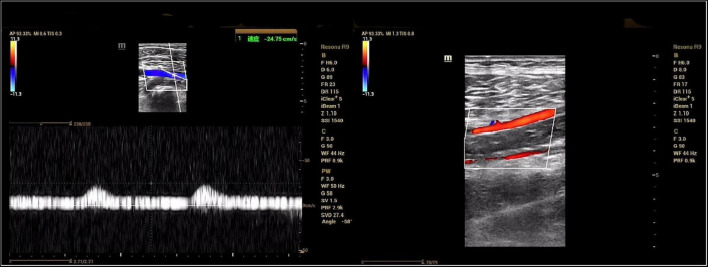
Preoperative ultrasonography. The spectral pattern was changed and triphasic waves were absent.
